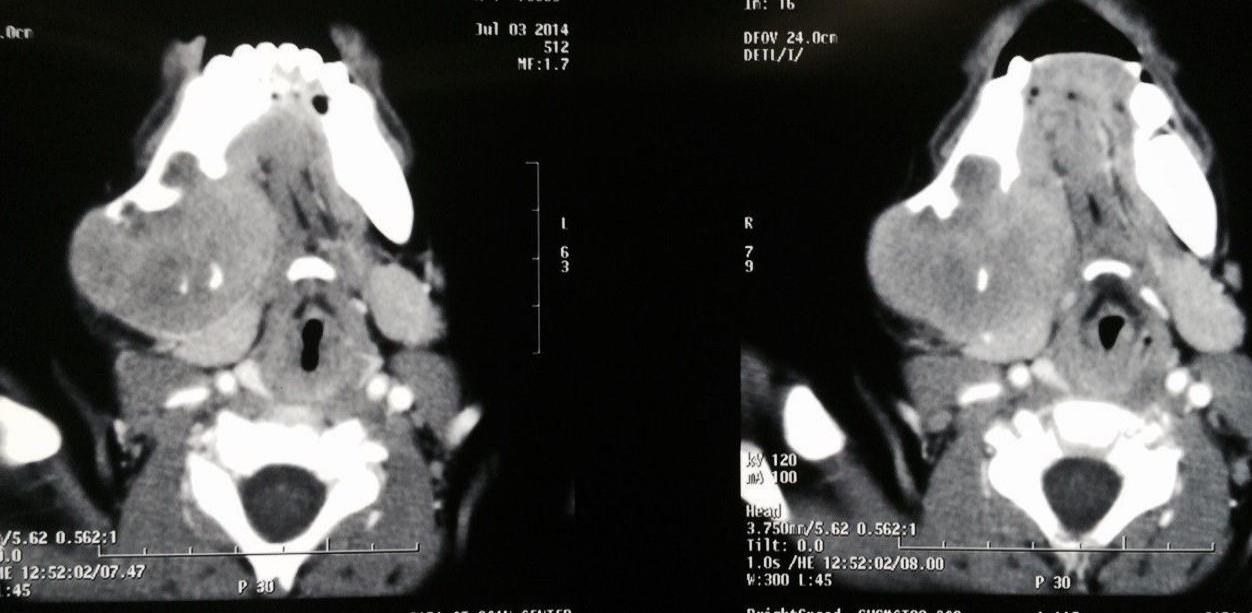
Axial CT scan of case 3 showing an expansile radiolucency in the right posterior mandible perforating the buccal and lingual cortices
